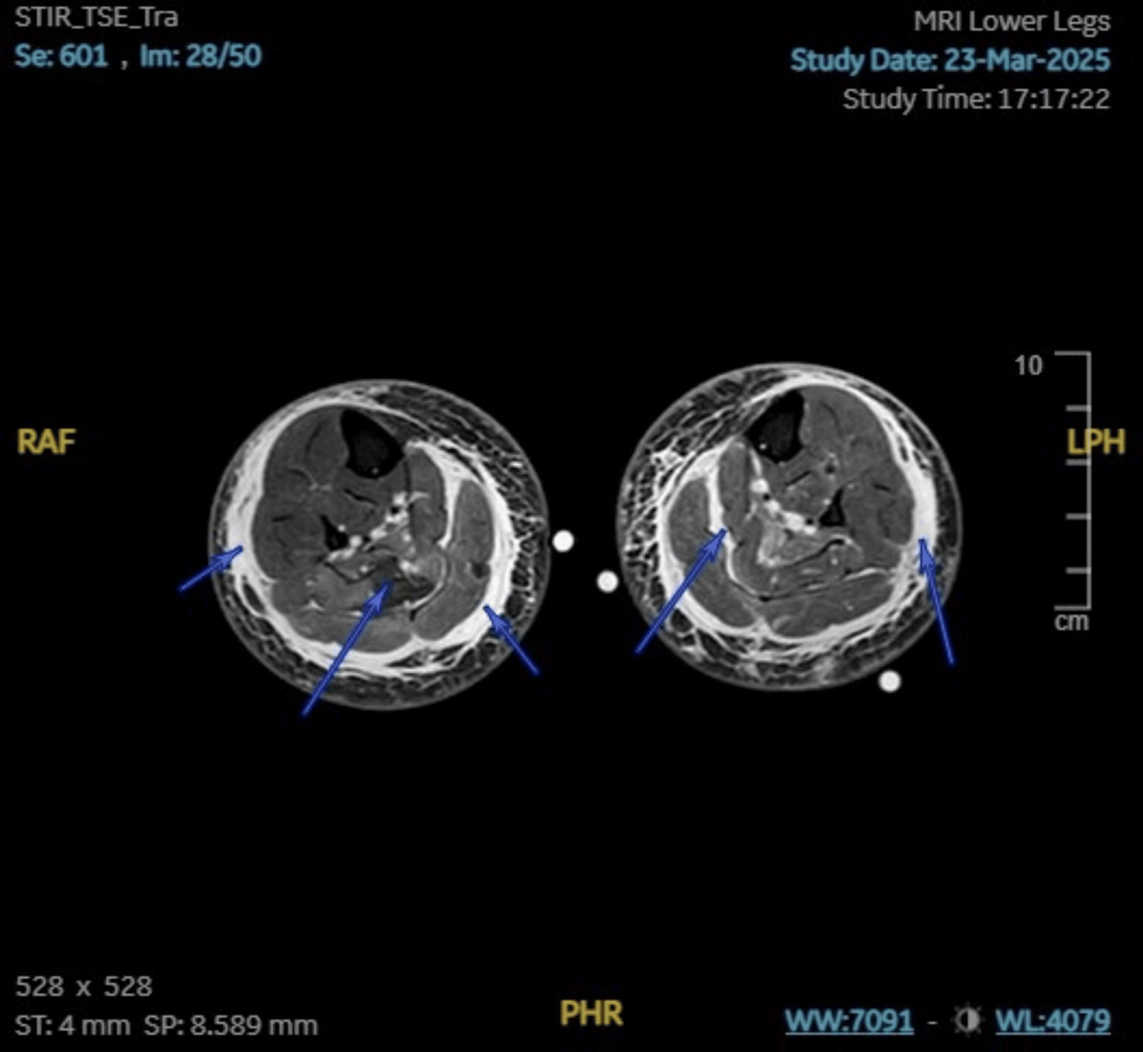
Axial short tau inversion recovery (STIR) view of the MRI of the lower limbs. Arrows indicate bilateral perimuscular and fascial soft tissue swelling of the lower limbs.
